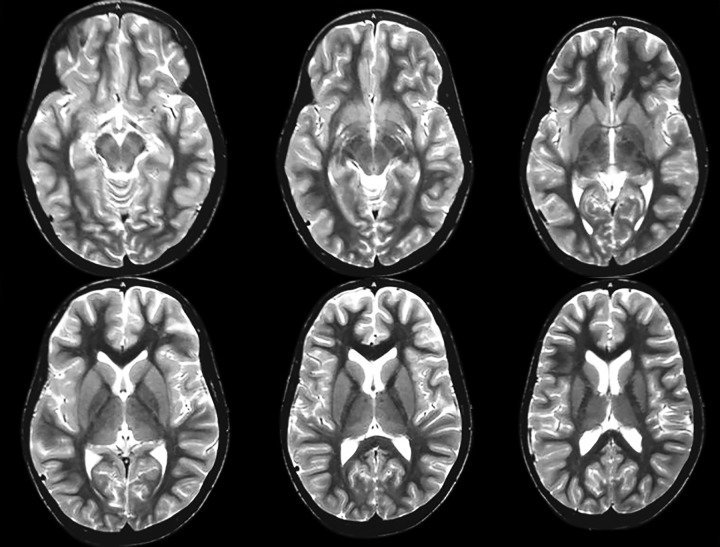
Magnetic resonance imaging revealed no obvious abnormality in the development and the condition of the brain
